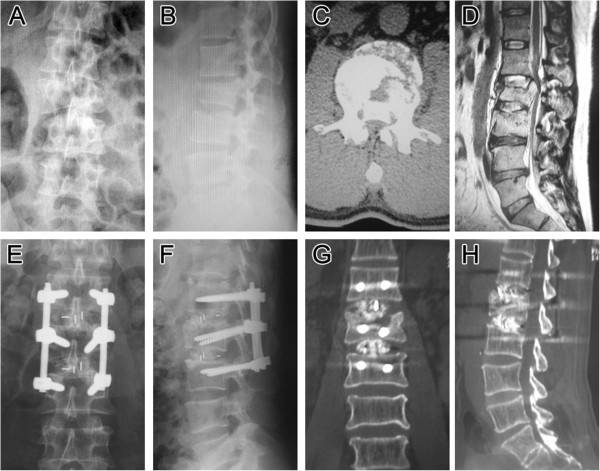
Follow-up images (case 18). Preoperative and postoperative imaging of a 45-year-old male patient (case 16) with L2 burst fracture, LSS _7, AO type A3.2, Danis type E with Frankel C neurologic impairment. A, B, Preoperative AP and lateral roentgenogram showing a L2 burst fracture with an angular segmental deformity of 17°. Preoperative axial CT scan image (C) and preoperative sagittal lumbar magnetic resonance imaging (D) demonstrating a significant spinal canal encroachment by retropulsion of the fragments of the fracture vertebra with laminar fracture. E, F, Postoperative AP and lateral plain radiograph showing a correct position of two cages placed to intervertebral space and the posterior transpedicular system. Angular deformity was corrected and segmental height was restored. G, H, Postoperative axial and sagital CT demonstrating a significant spinal canal clearance and satisfied position of the cages and normal thoracolumbar alignment and some of the grafted bone chips was inserted into the vertebral body through the fractured endplate.
